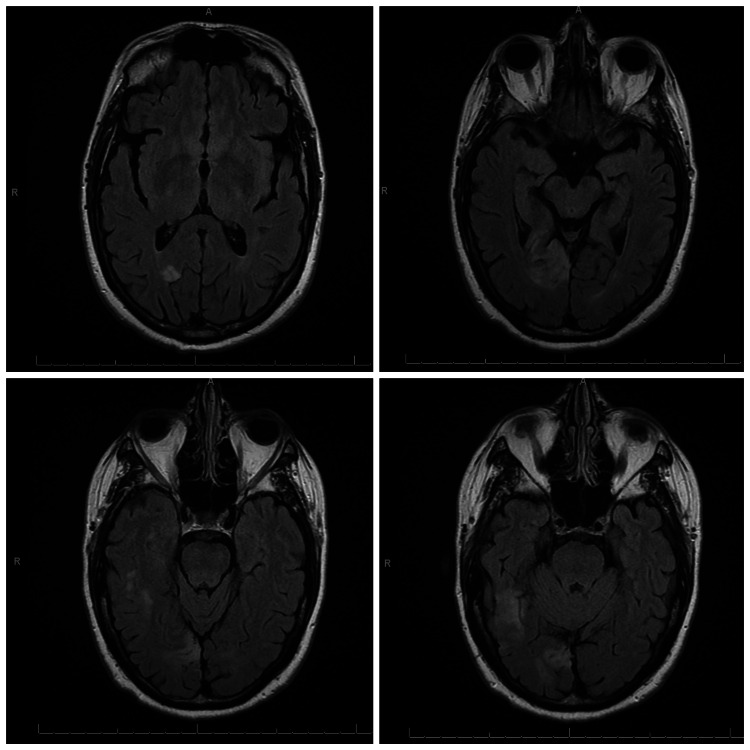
T2-weighted MRI sequence showing increased signal intensity and vasogenic edema in the right occipital lobe and right posterior temporal lobe region MRI: magnetic resonance imaging
